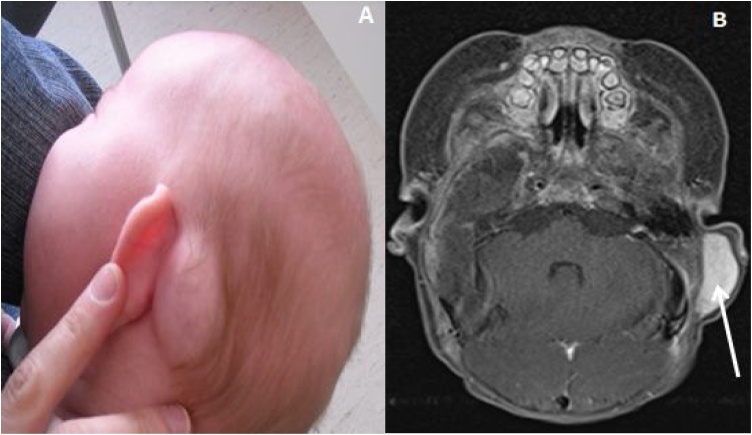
Clinical photograph of Patient #1, with the left retro auricular primary lesion (A) confirmed with axial post contrast MRI of head, fat-saturated, T1-weighted showing moderately intense (white arrow) and heterogeneous enhancement of the mass (B).
